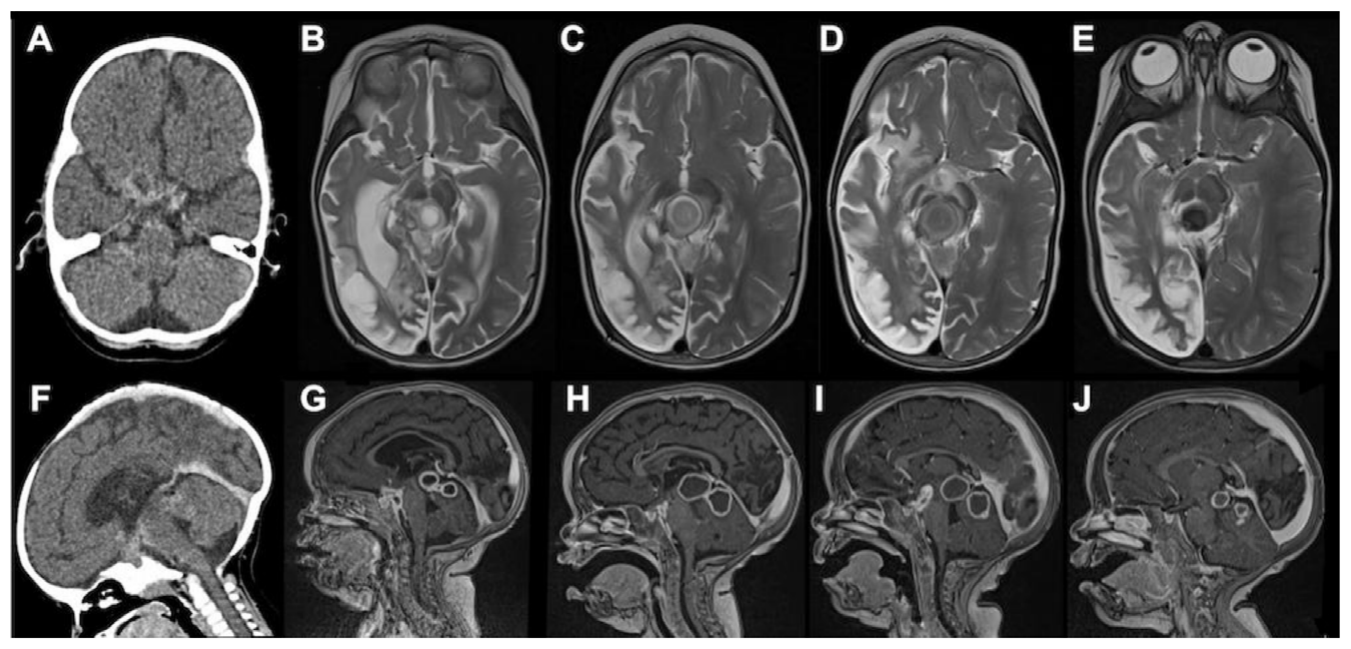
CT axial, MRI T2 axial, CT sagittal and MRI T1 post-gadolinium sagittal images at 3–4 month intervals of a 16-month-old HIV-infected female with stage III TBM. The initial CT axial and sagittal scans (
